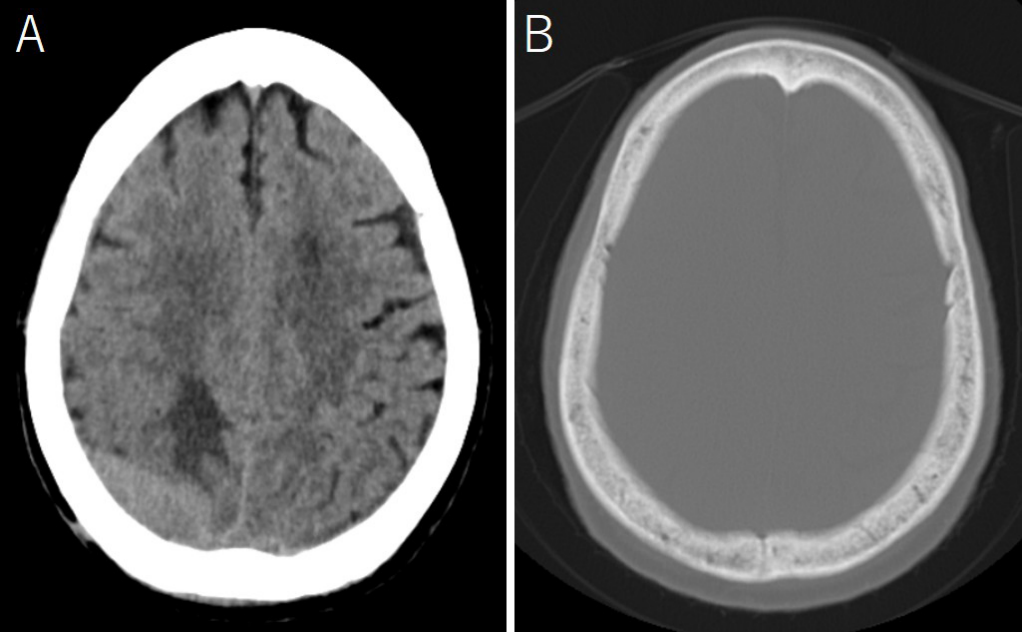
CT showed a slightly high-density mass in the right parieto-occipital region with peritumoral edema (A). The bone window view showed no signs of invasion by the tumor (B). CT: computed tomography.
